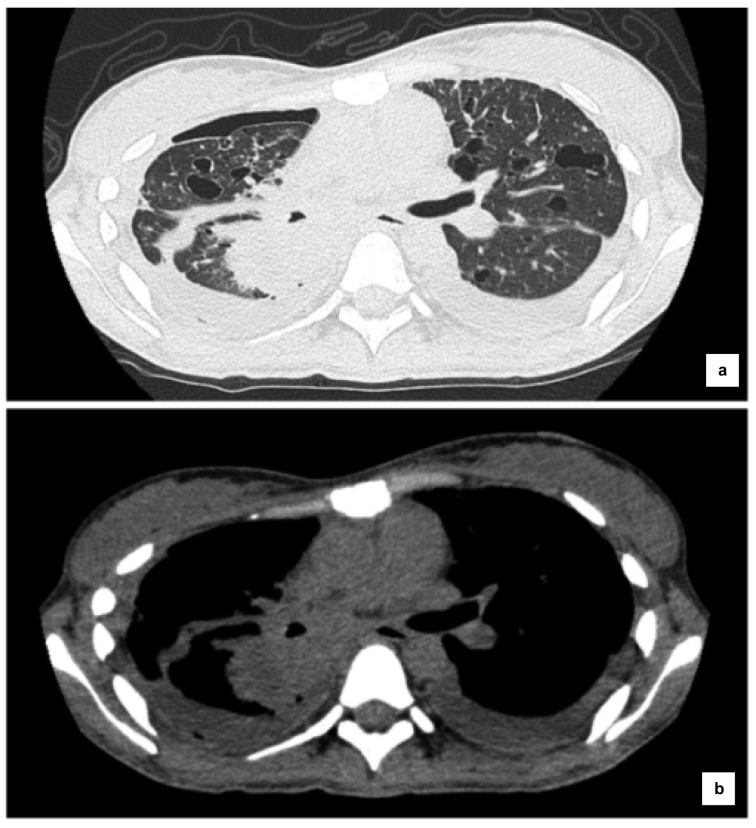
Computed tomography (CT) scan before administration of osimertinib. Primary lesions in the right middle and lower lobes, multiple cystic lesions in the lung field, and mild pneumothorax were observed in the right lung (a). Pleural effusion on both sides was observed (b).
